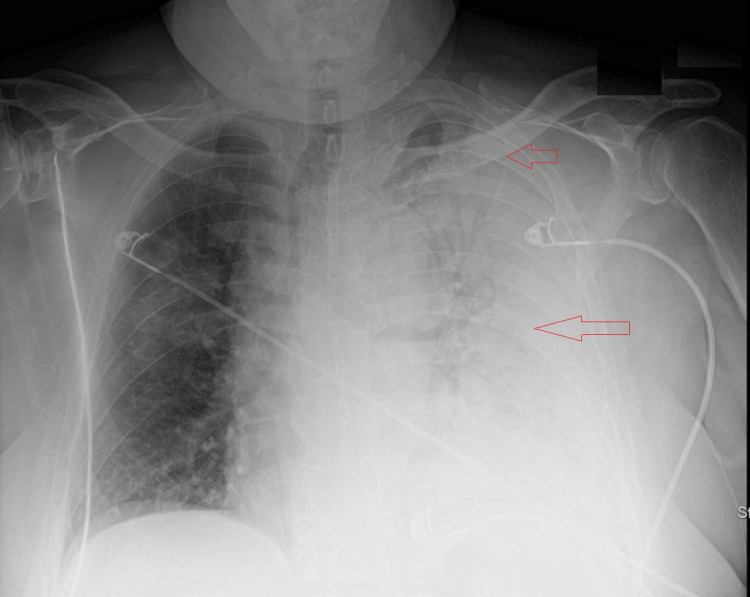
Chest X-ray demonstrates opacification of the entire left hemithorax with associated air bronchograms.
